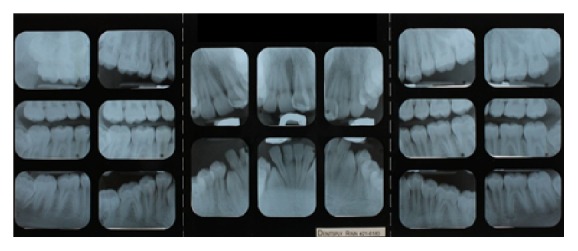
Initial full mouth radiographs.
